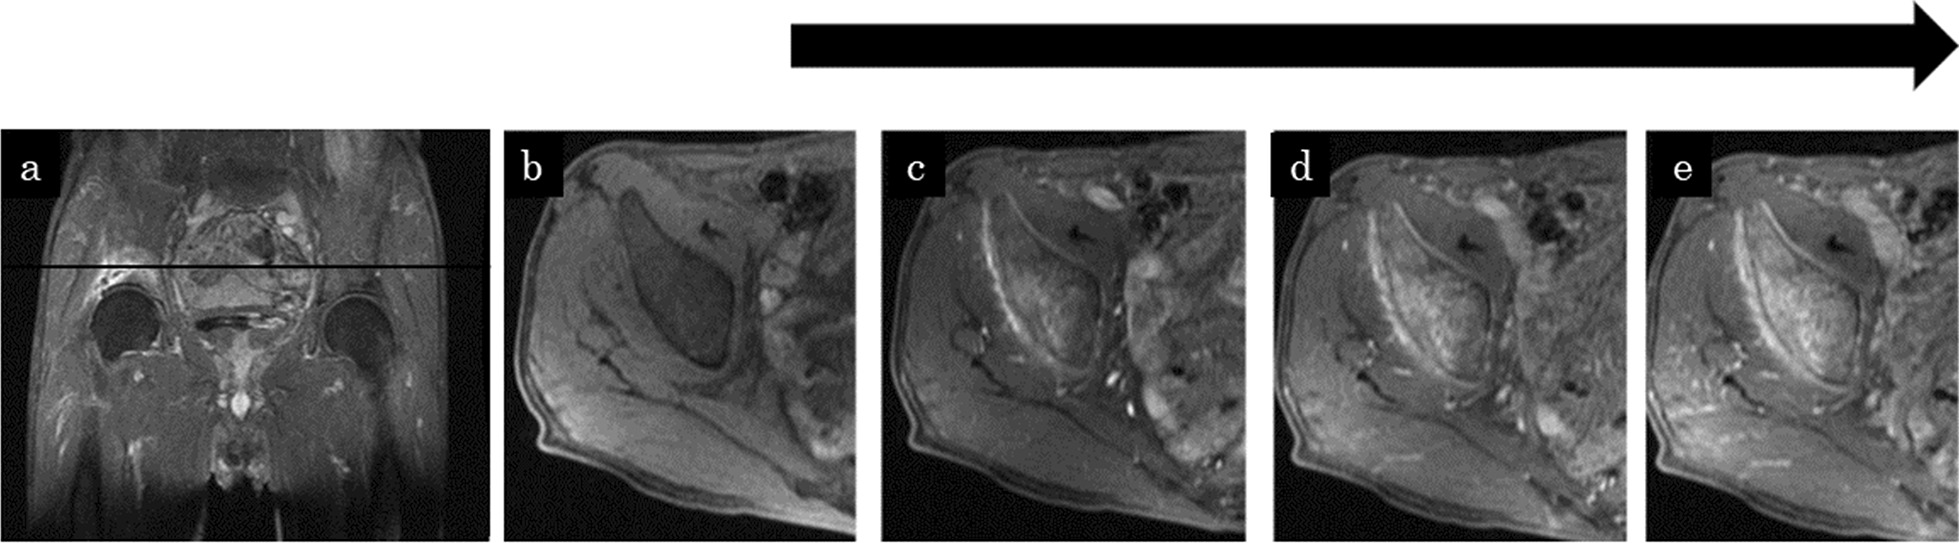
a Contrast-enhanced MRI, coronal plane. Black line indicates the level of the axial plane (b–e). b Pre-contrast-enhanced MRI, axial plane. c–e Contrast-enhanced MRI, axial plane. Black arrow indicates time passing after administration of contrast medium. They showed the lesion gradually enhanced
